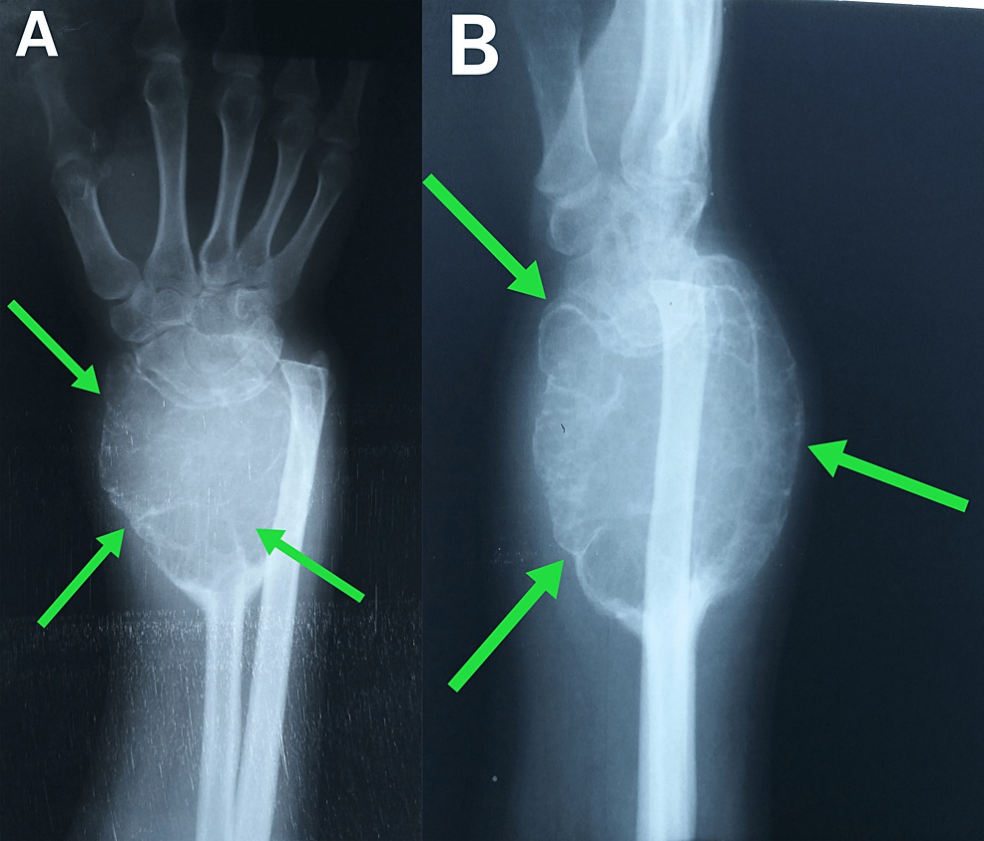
Pre-operative anteroposterior (A) and lateral (B) X-rays of the wrist. The arrows indicate a huge lobulated lesion that invades the distal third of the radius and surrounds the dorsal and palmar aspects of the proximal row of the wrist bones. The lesion causes medial dislocation and elongation of the ulna.
